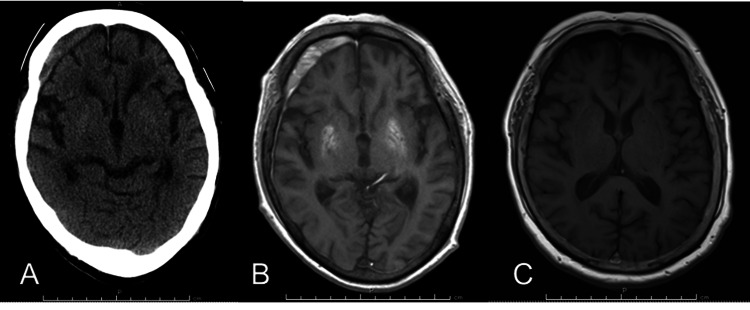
Brain images CT scan of the head at admission showed a subdural hematoma in the right frontal region with no abnormalities in the basal ganglia (A). T1-weighted MRI of the head at the time of admission. High-signal areas were seen in the bilateral putamen (B). T1-weighted MRI image of the head three years before admission. There is no high-signal area in the basal ganglia (C).
